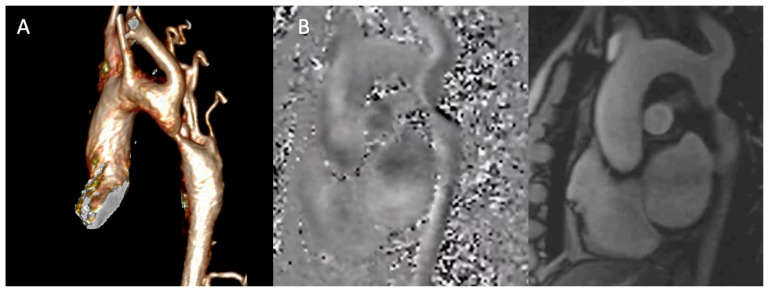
(A). CE-MRA 3D volume rendering reconstruction of aortic coarctation. (B) Phase contrast MRI in sagittal view of aorta.
